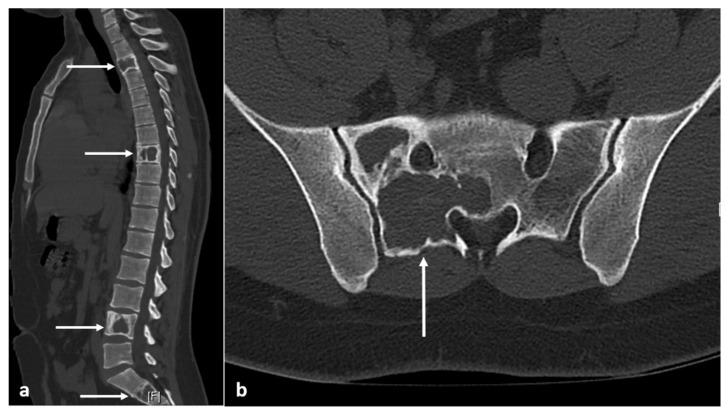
Sagittal (a) and axial (b) CT images demonstrating fibrous dysplasia of thoracic and lumbar vertebrae and sacrum (white arrows). The ground glass matrix is not always seen, and some such as this can appear lytic with thin peripheral cortical shell.
